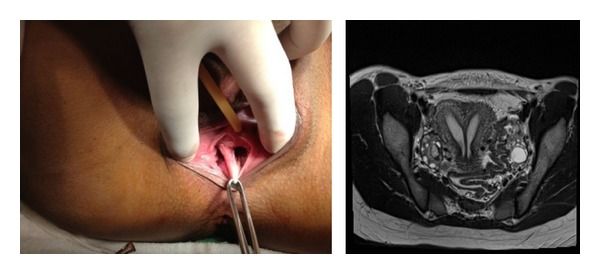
Complete septum evidenced two vaginal cavities (left). Pelvic image of magnetic resonance imaging showing septate uterus (right).
